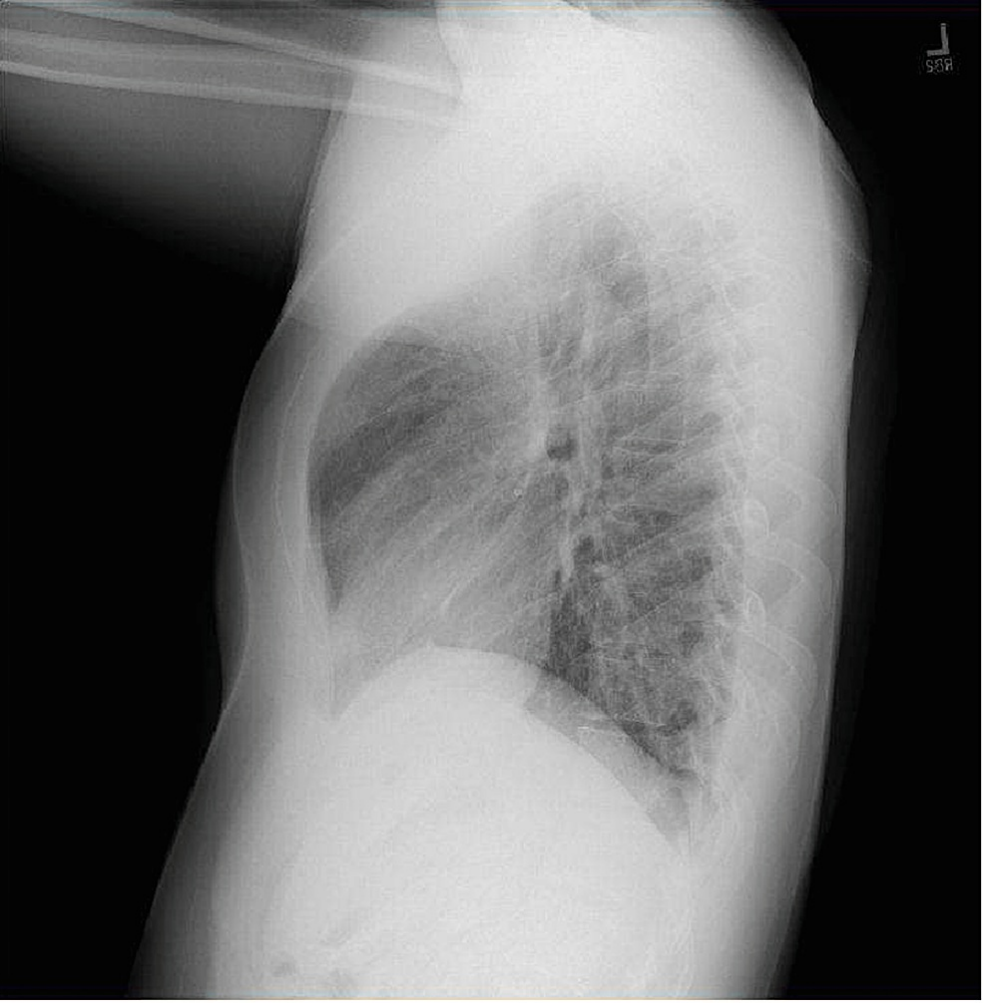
Follow-up anteroposterior and lateral radiographs at two-week follow-up visit demonstrating sustained resolution of the pneumothorax
